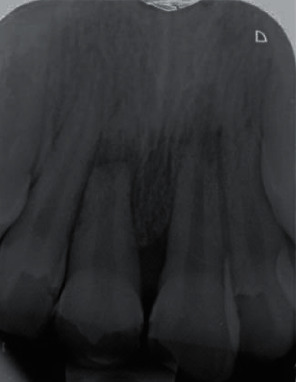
Periapical radiographs of the maxillary central incisors.
